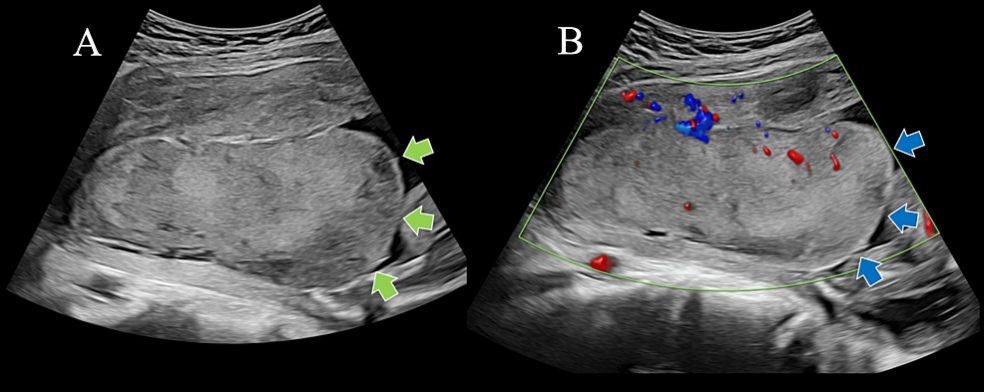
Changes in echogenicity of the placental mass (A) A placental mass (green arrow) with confirmed low echo density on ultrasound at 23 + 0 weeks of gestation. (B) A placental mass (blue arrow) with changed echogenicity at 23 + 2 weeks of gestation.
